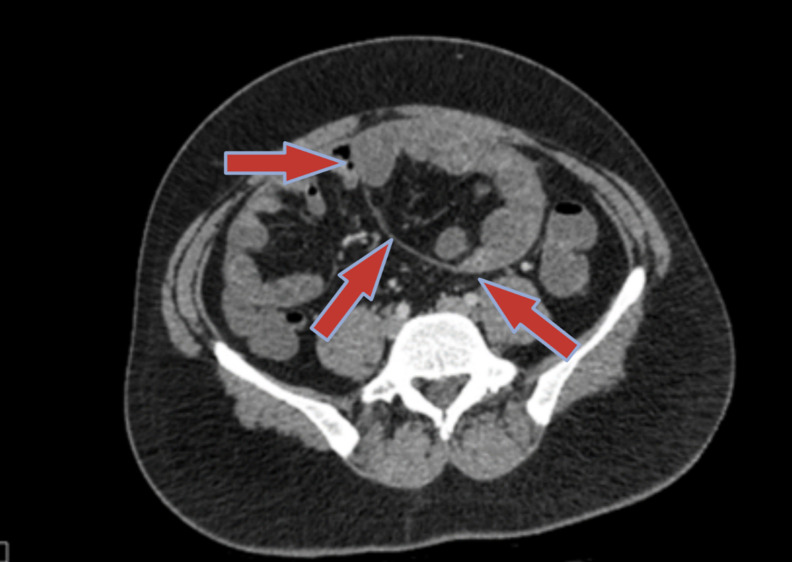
Computed tomography enterography shows edematous small intestines and fibrous tissue surrounding them, similar to those detected in ultrasonography.
